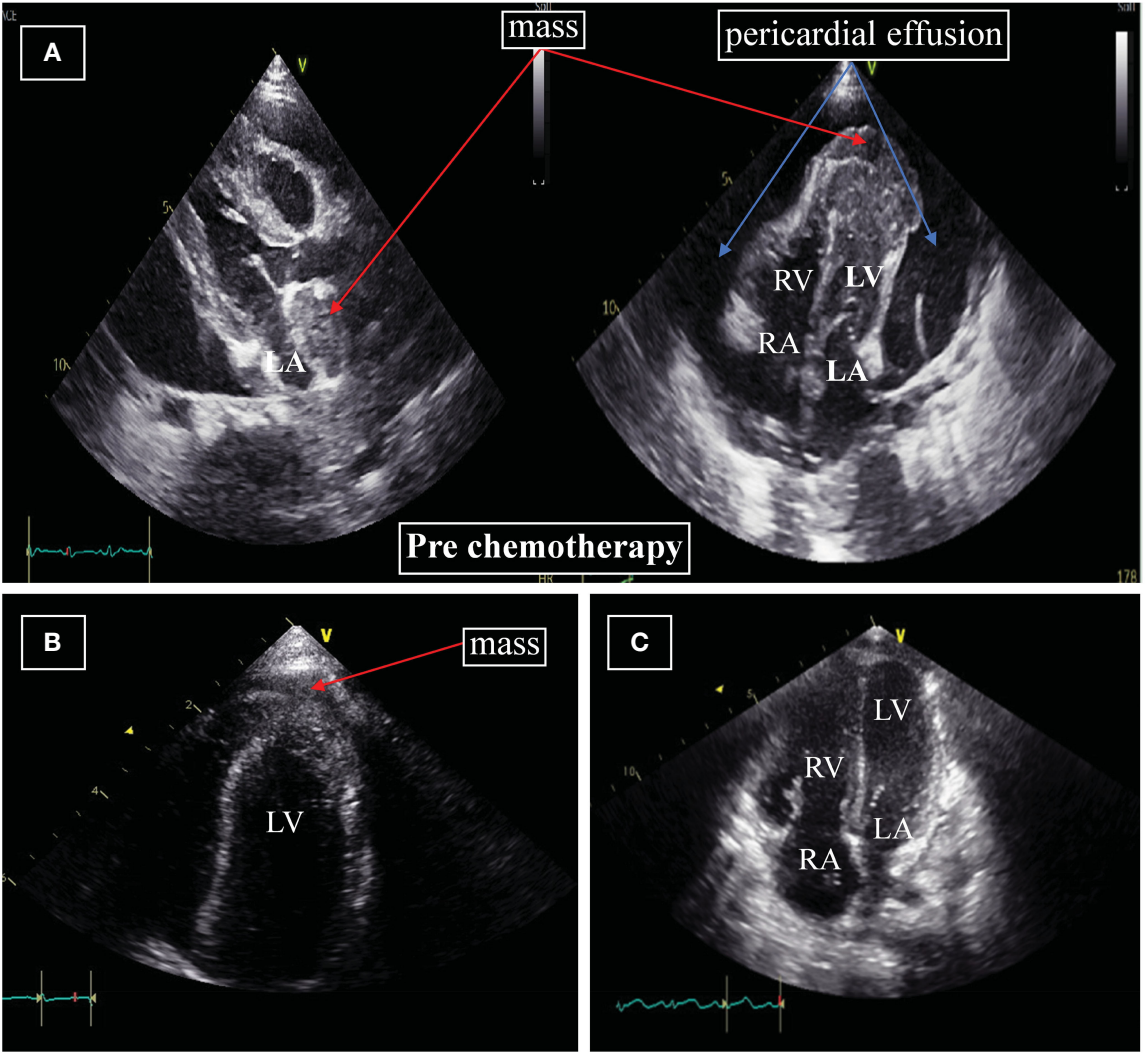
(A) Echocardiography at diagnosis. The apex of the left ventricle and left atrium displayed mass echoes protruding into the pericardial cavity, measuring 3.7×3.4cm and 2.3×1.2cm, respectively. (B) Echocardiography after four weeks of initiation of systemic chemotherapy. Significant reduction in the size of the mass to 2.2×0.7cm; the mass on the apex of the left atrium and pericardial effusion are no longer displayed. (C) Echocardiography after two months. The masses around the left ventricle and left atrium, pericardial effusion are not displayed. LA=left atrium; RA=right atrium; LV=left ventricle; RV=right ventricle.
